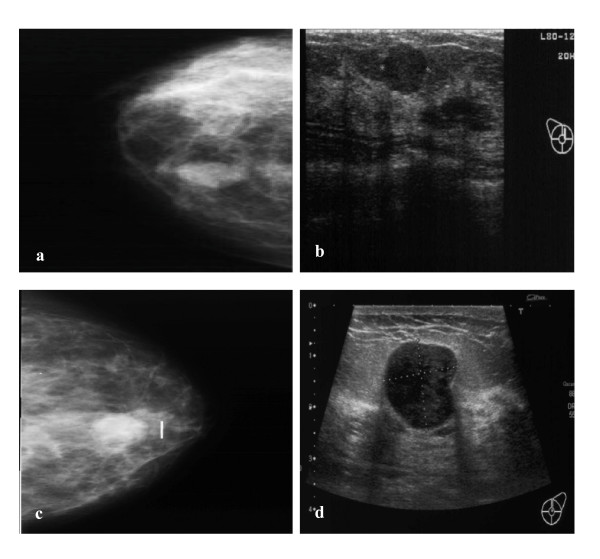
Radiology images from 2 cases presenting with a breast mass. a. Case 1. Mammogram of right breast. b. Case 1. Ultrasound of right breast. c. Case 2. Mammogram of left breast. d. Case 2. Ultrasound of left breast.
